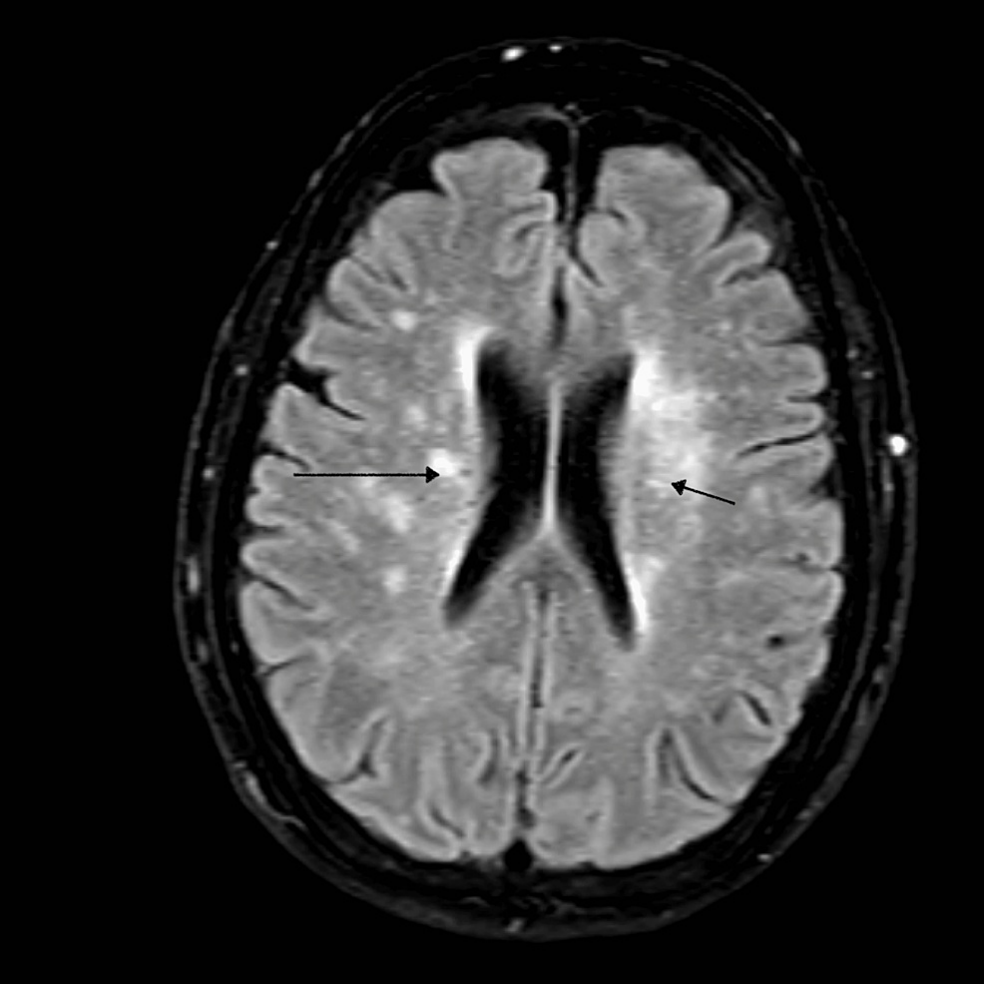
MRI Brain showing multiple embolic infarcts (arrows)
